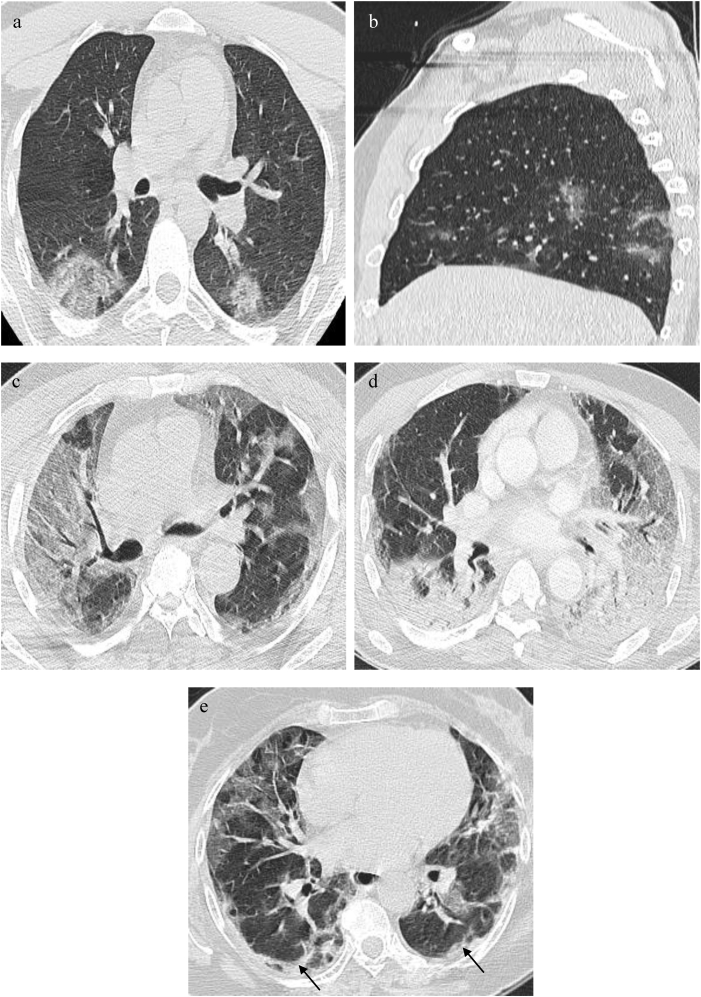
Covid-19 pneumonia's chest CT imaging features' changes over time.*(a,b) Early stage: Ground glass opacities involving the lower lobes with partial crazy paving;(c) Progressive stage: Ground glass opacities extension and increased crazy paving;(d,e) Peak stage: Consolidative opacities, sub-pleural lines (Black arrow) and bronchiectasis.*
